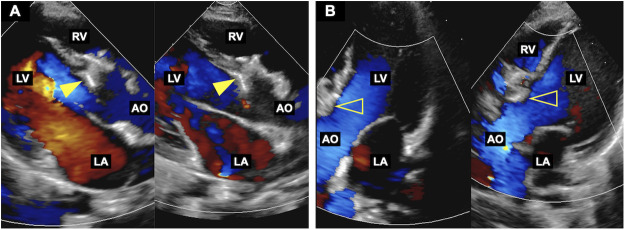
Cyclic deformation of the left disc skirt on serial echocardiography. (A) During diastole, the left disc skirt (arrowhead) is compressed by the posterior excursion of the right coronary cusp. (B) During systole, the skirt (open arrow) re-expands, restoring its normal contour.
